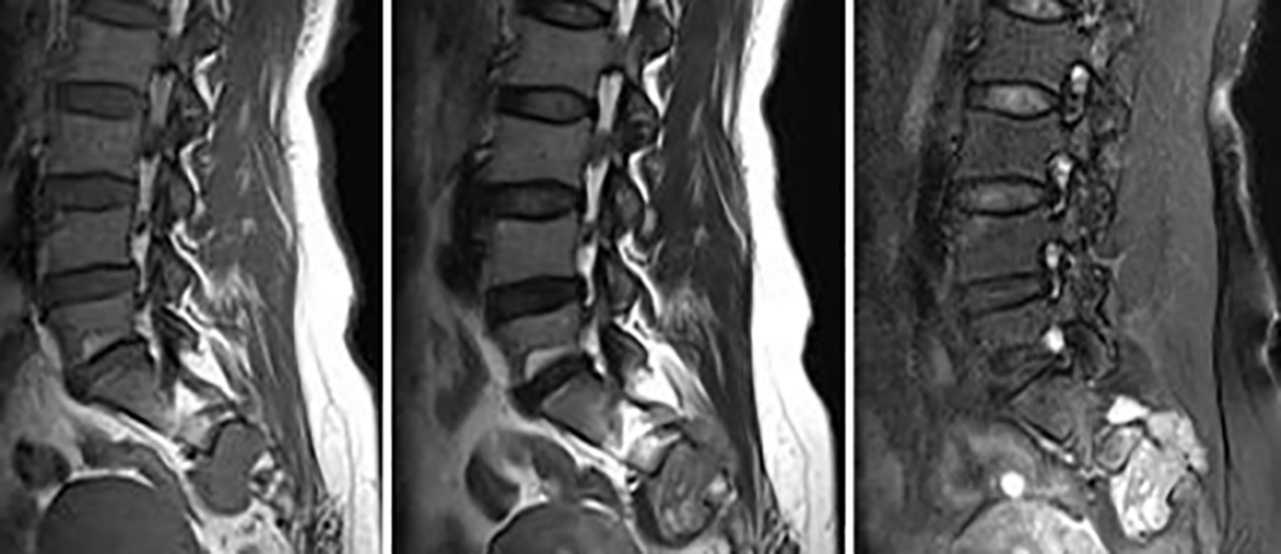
Primary spinal epithelioid hemangioendothelioma (EHE) in a 42-year-old woman. A sagittal image of the lumbar spine reveals a mass in the sacral 2–4 vertebrae with a paravertebral soft tissue mass. MRI showed an inhomogeneous low signal on T_1_-weighted image (T_1_WI) and a high signal on T_2_WI with punctate low-signal foci. Contrast-enhanced MRI demonstrated intensive inhomogeneous enhancement of the lesions with punctate low-signal foci.
